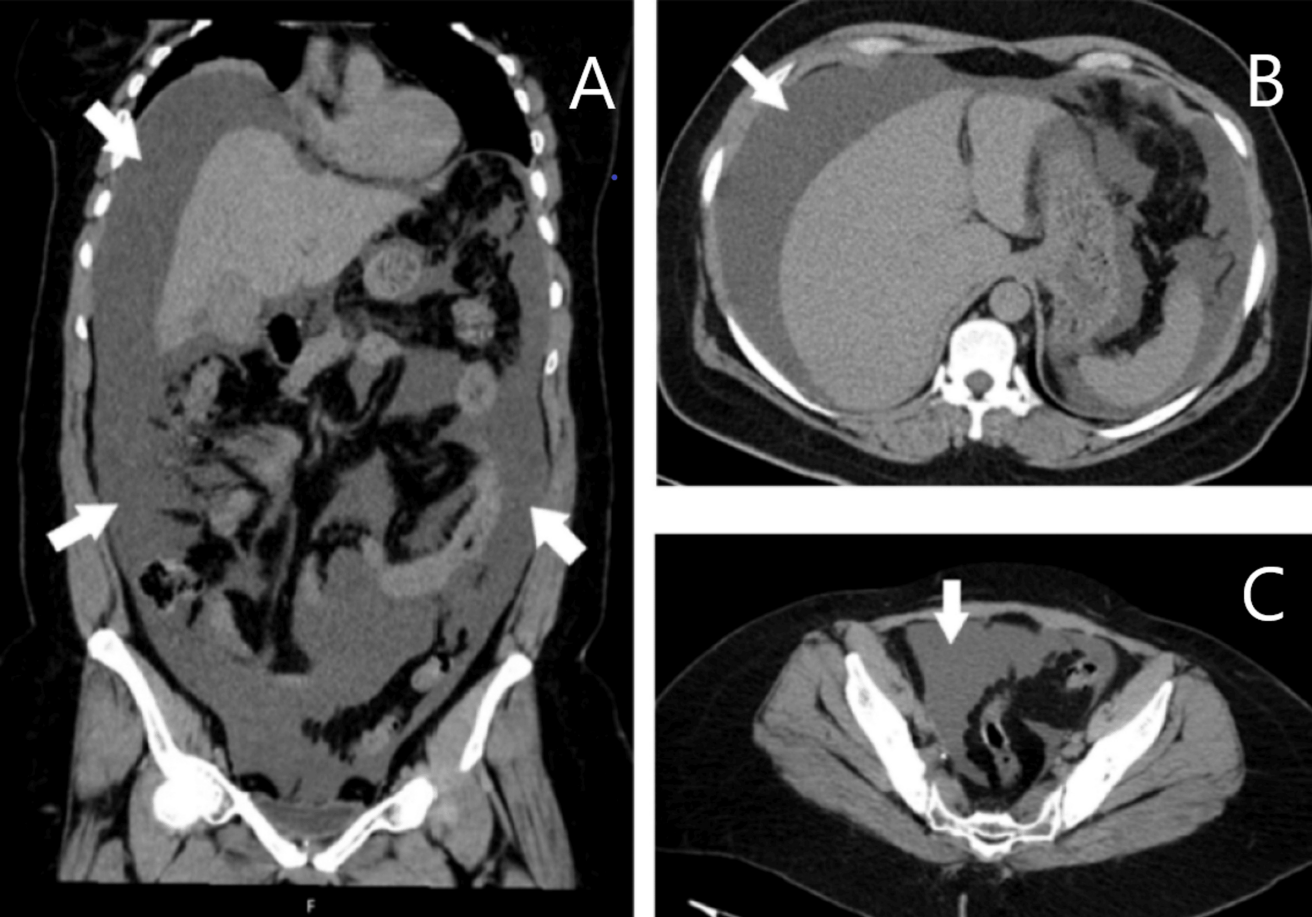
CT scan findings on admission Abdominal CT showing moderate-to-large-volume ascites (marked by the white arrows). Sub-image A displays the coronal plane. Sub-images B and C depict the axial plane.
